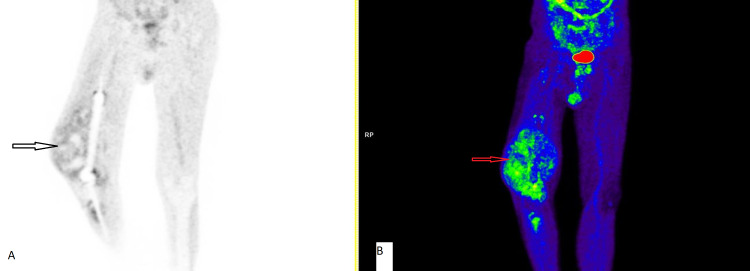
Positron emission tomography–computed tomography showing an orthopedic prosthesis in the knee region with a large lobulated fluorodeoxyglucose (FDG) avid lesion involving the right knee joint region with areas of necrosis and calcification. Seen with Maximum Intensity Projection (MIP) black & white image (A)  and colored image (B).
